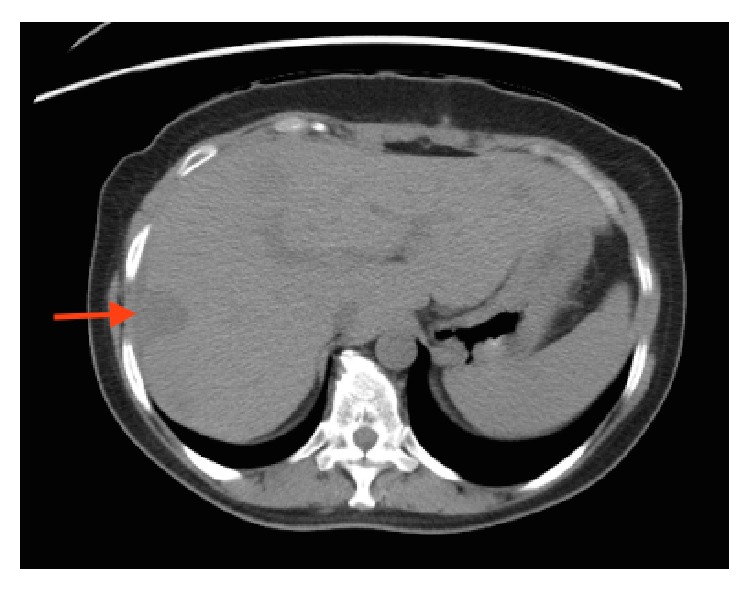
Axial section of CT scan showing the nodule in the liver which represents benign leiomyomatous nodule (red arrow).
